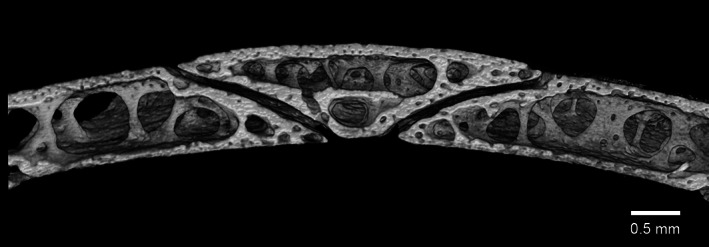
Micro‐CT of the ossicle ring of a gentoo penguin chick (*Pygoscelis papua*, G2) showing one ossicle overlapping two others. There are spaces within the still developing bone of the chick, not present in any adult examined, yet the scleral ring is clearly already present at 10 weeks
